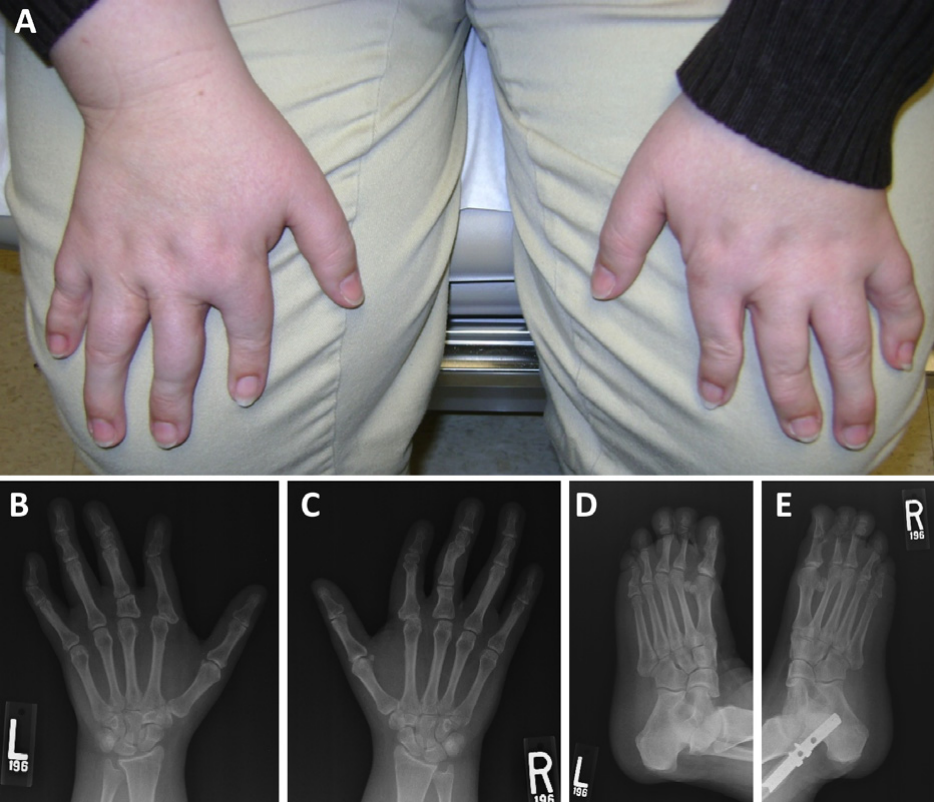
(A) At present, the hands of the proband are notable for malformed digits. (B and C) X-rays of the hands at present. *See text for detailed description*. (D and E) X-rays of the feet at present. *See text for detailed description*.
